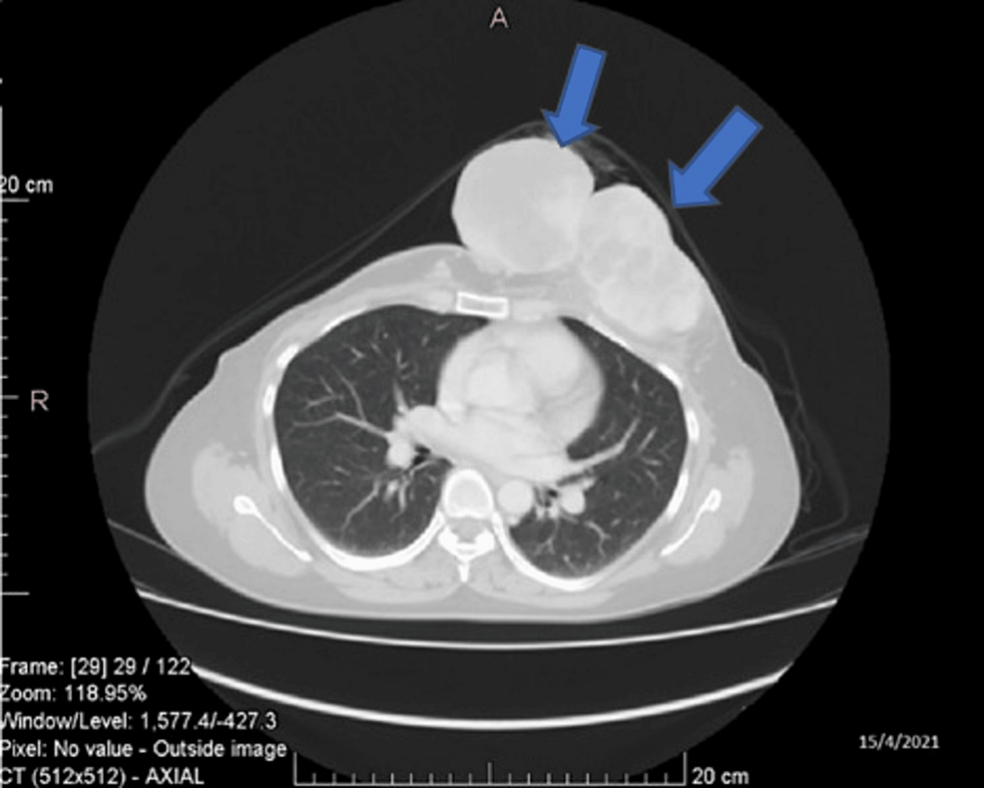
CT imaging (taken April 2021) demonstrated a left anterior chest wall mass (blue arrows).
